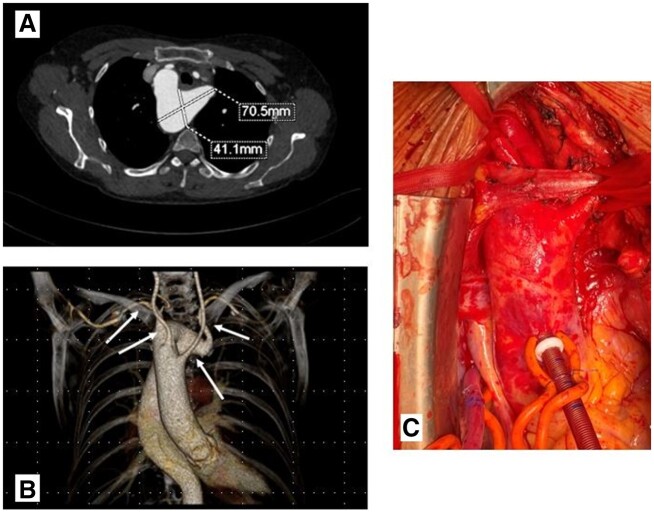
(A) Contrast computed tomography of Kommerell’s diverticulum, (B) computed tomography three-dimensional reconstruction: 4 vessels arising individually and (C) intraoperative anatomy: looped right subclavian artery, right common carotid artery, left common carotid artery and brachiocephalic vein.
